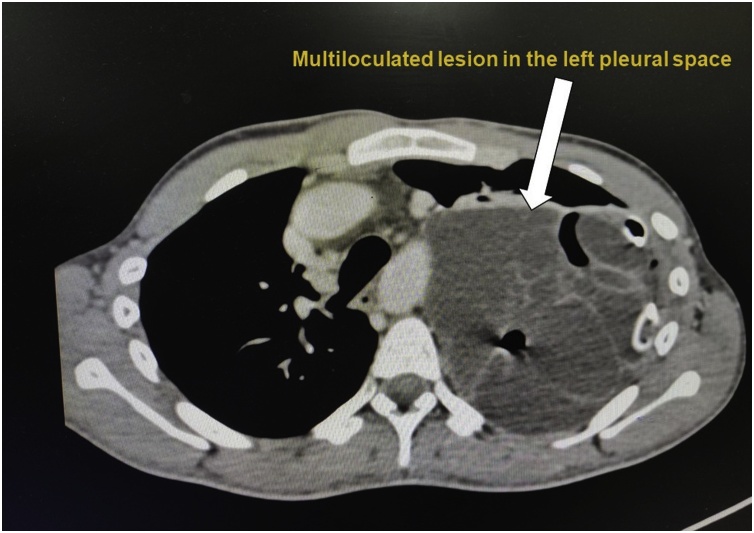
PET CT done in February 2018 showed “appearance of multiloculated lesions in left pleural space as a new finding”.
